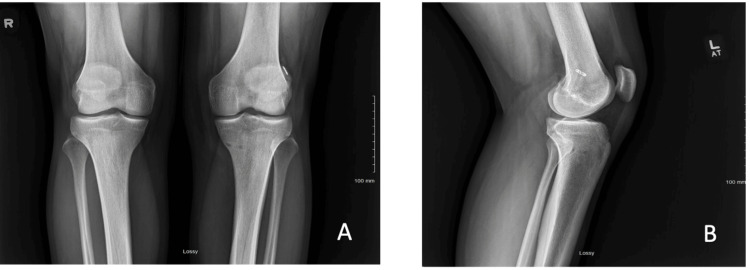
Three-month post-operative x-rays. (A) Anterior-posterior views of the knee. (B) Lateral view of the knee.
